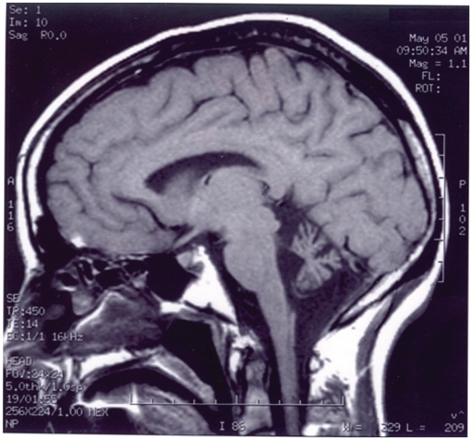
MRI of Patient with the p.Arg423His mutation. Cerebellar atrophy in a 48 year old female (H2591) with ataxia and the KCNC3^Arg423His^ mutation. Midsagittal T1-weighted MRI of the brain shows a small atrophic cerebellum with a normal appearing brainstem.
